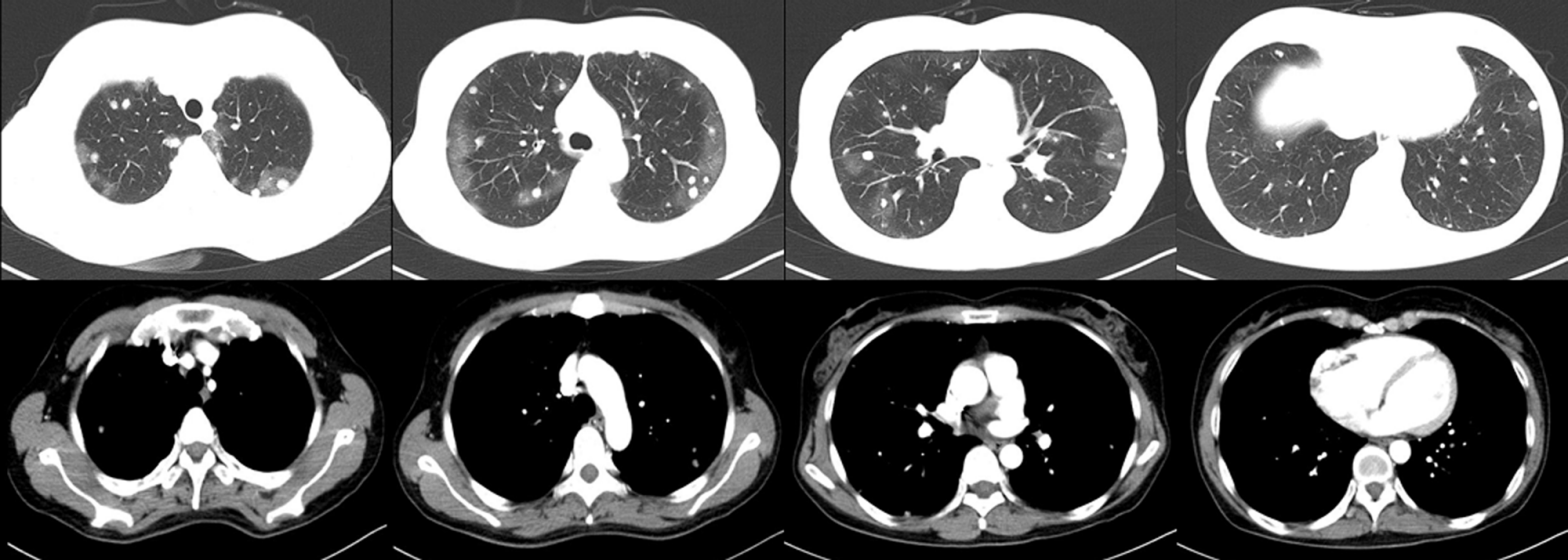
Chest CT on August 26, 2018. Re-examined chest CT showed more and larger nodules in both lungs than previously.
